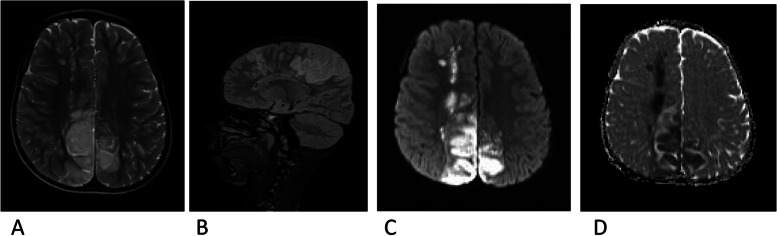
MR images axial T2W (A), sagittal FLAIR (B), axial DWI&ADC (C&D) revealed territories in the right frontal and both parietal lobes involved with acute watershed infarcts. Abbreviations: FLAIR- Fluid-attenuated inversion recovery, DWI diffusion-weighted imaging, ADC apparent diffusion coefficient map
